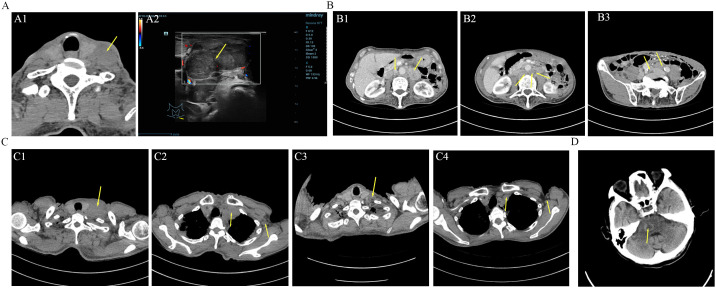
CT and ultrasound findings of lymph node metastases and brainstem involvement. (A) Left supraclavicular lymph node at diagnosis (CT, A1; ultrasound, A2). (B) Abdominal CT showing retroperitoneal and para-iliac lymph node metastases (B1-B3). (C) CT comparison before (May 2025; C1-C2) and after treatment (August 2025; C3-C4). (D) Brain CT showing brainstem metastasis (December 2025). CT, computed tomography.
